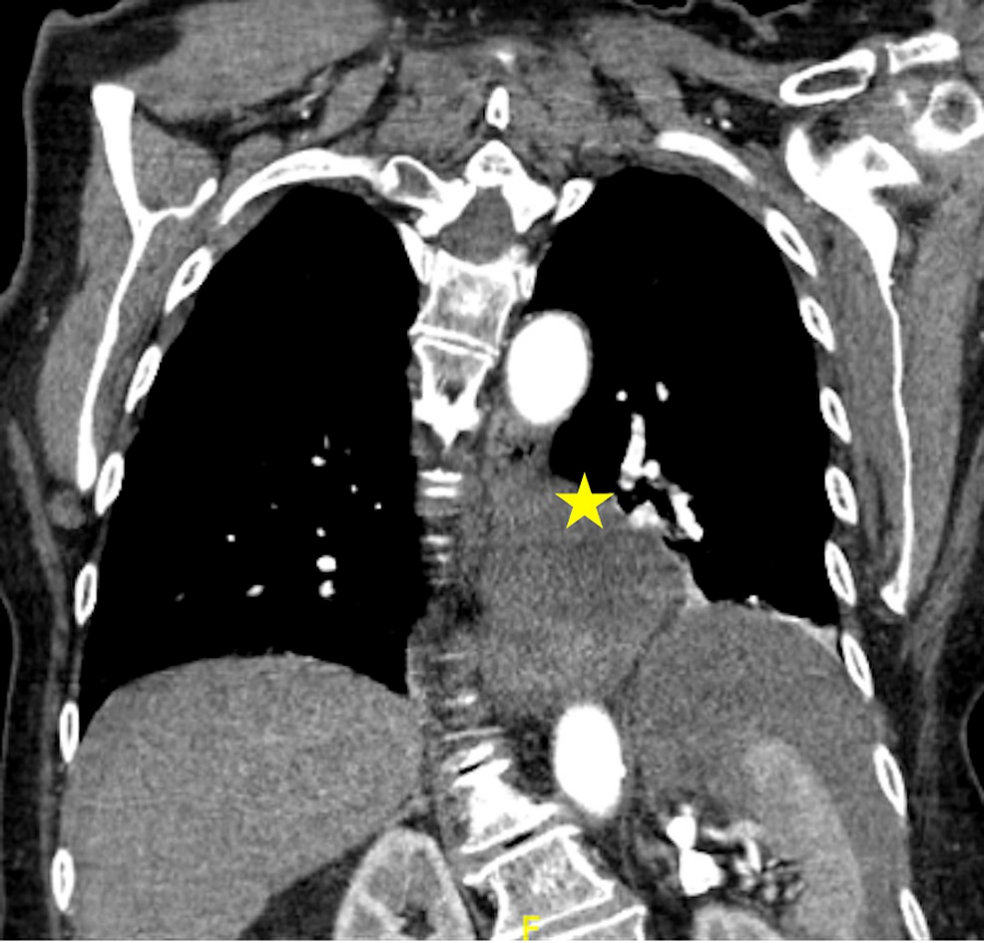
CT with contrast angiography with a coronal view of a large hiatal hernia (star) with suspected organo-axial volvulus and free fluid surrounding the distal esophagus with moderate hemoperitoneum surrounding the liver and spleen
